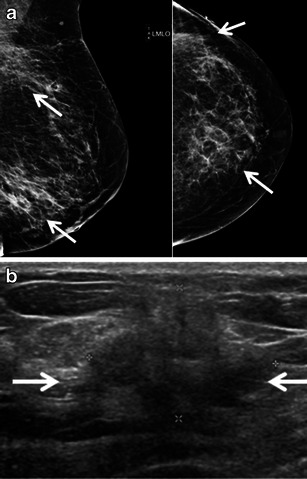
An 83-year-old woman with known acute T-cell prolymphocytic leukaemia presents with new palpable masses in the left breast. a Left medio-lateral oblique and cranio-caudal diagnostic mammogram demonstrates two areas of architectural distortion corresponding to the two palpable masses (*arrows*). b Ultrasound shows ill-defined mixed echogenic and hypoechoic mass (*arrows*). The other palpable mass had a similar sonographic appearance (*not shown*)
